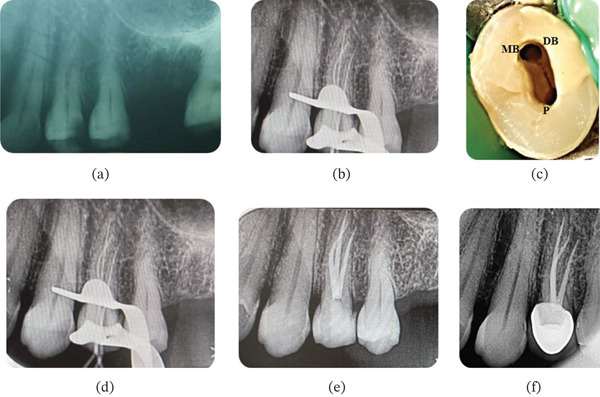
(a) Preoperative radiograph in relation to 24; (b) working length radiograph; MB = 20.5 mm, DB = 19.5 mm, and P = 22 mm; (c) T‐shaped access cavity showing three distinct canal orifices, with the MB and DB orifices located in close proximity under magnification; (d) radiographic confirmation of master cone; (e) obturation and core build up radiograph; and (f) 1 year follow‐up radiograph with post endodontic PFM full coverage crown.
